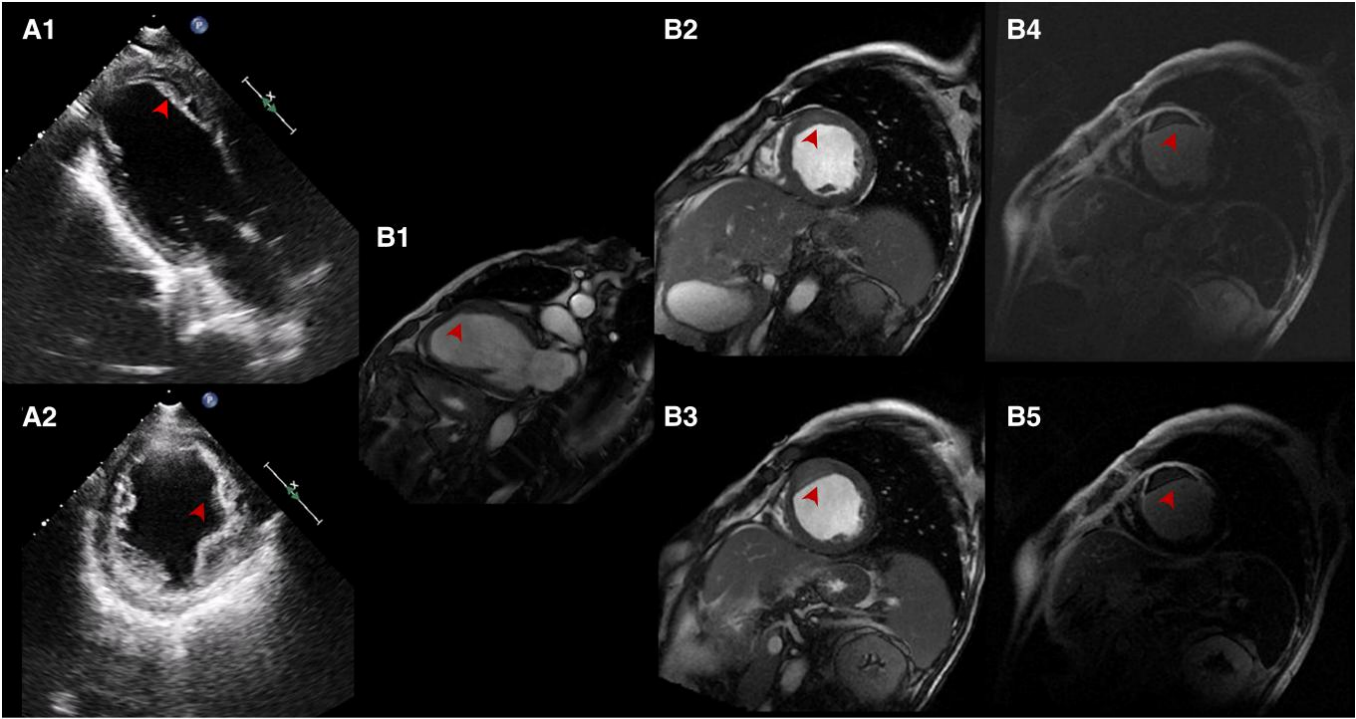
Multimodality cardiac imaging in Patient 1. TTE apical two-chamber (A2C) (*A1*) and short-axis (SA) (*A2*) views show a flap-like endocardial border separation compatible with an IMDH (arrowheads). CMR balanced steady-state free precession (bSSFP) cine A2C (*B1*) and SA (*B2* and *B3*) sequences early after gadolinium contrast administration: note that the apical semilunar shaped IMDH (arrowheads) is separated from the LV, by a ‘flap-like’ structure. LGE SA images (*B4* and *B5*) demonstrate hypointense and surrounded by hyperintense myocardium at the anterior and apical cavity as well as transmural infarction (arrowheads). Note that the aforementioned ‘flap-like’ structure appears as a thin hyper-enhanced layer corresponding to the endocardial border.
